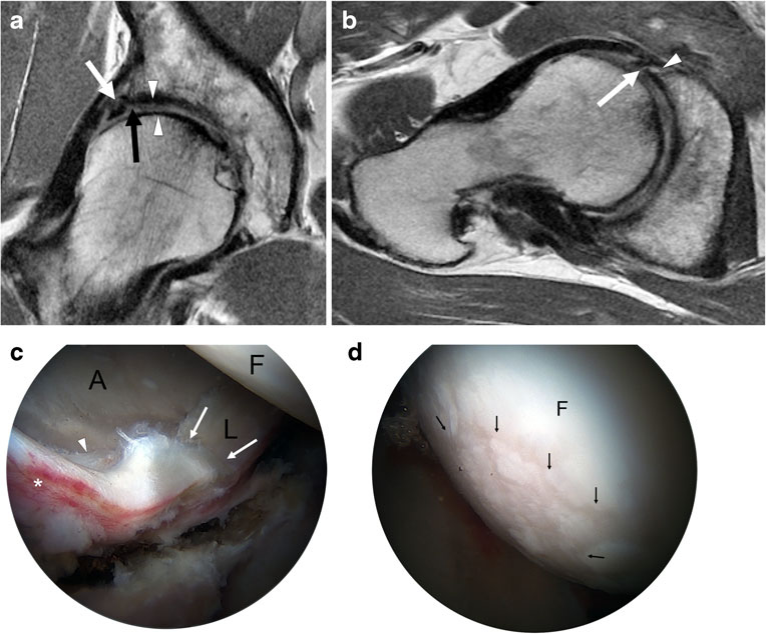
A 48-year-old man with combined cam and pincer impingement. a Coronal PD-weighted MRI demonstrates labral degeneration with intralabral ossification (*white arrow*). Separation is seen at the chondrolabral junction (*black arrow*). There is moderate to high-grade chondral wear over the superior femoral head and anterosuperior dome (*arrowheads*). b Axial oblique PD-weighted image shows a non-displaced tear of the anterior and anterosuperior labrum (*arrow*), with an associated intralabral cyst (*arrowhead*). c Arthroscopy image in the same patient demonstrates the labrum (L), acetabular (A), and femoral head (F) articular surfaces. An intrasubstance labral tear between 12 and 4 o'clock (*white arrows*), impaction erythema (*asterisk*), and chondral delamination at the transition zone (*arrowhead*) are seen. d There is moderate chondral wear (*black arrows*) over the femoral head, and normal cartilage is seen adjacent to this area (F)
